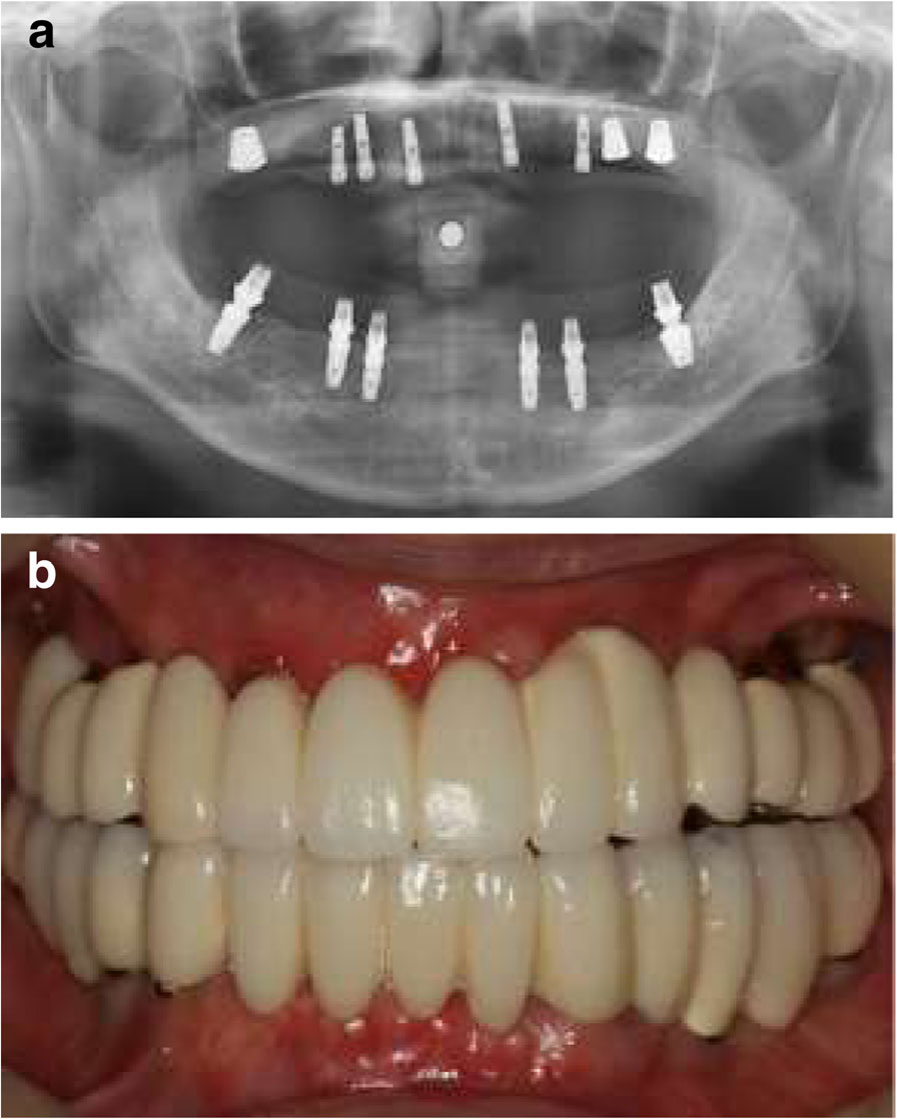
Patient after full-arch rehabilitation with immediately loaded implants. a Panoramic radiograph after surgery b Final implant supported prosthesis
